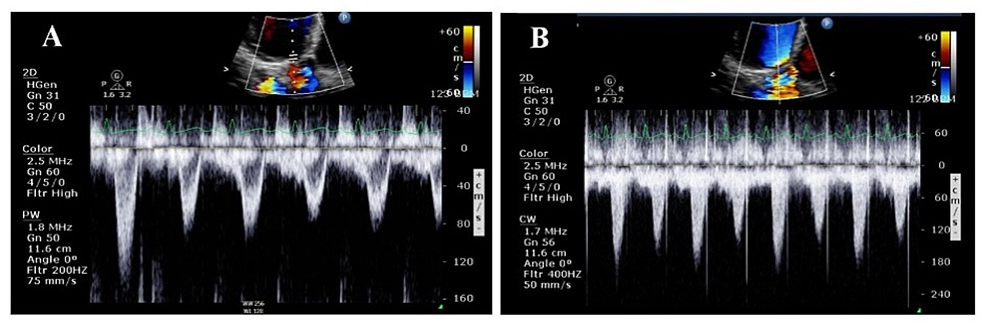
Postoperative echocardiogram. Pulse-wave Doppler of the left ventricular outflow tract (A) and continuous-wave Doppler of transaortic flow velocity (B) show significant beat-to-beat variation of stroke volume and peak velocity, consistent with pulsus alternans.
